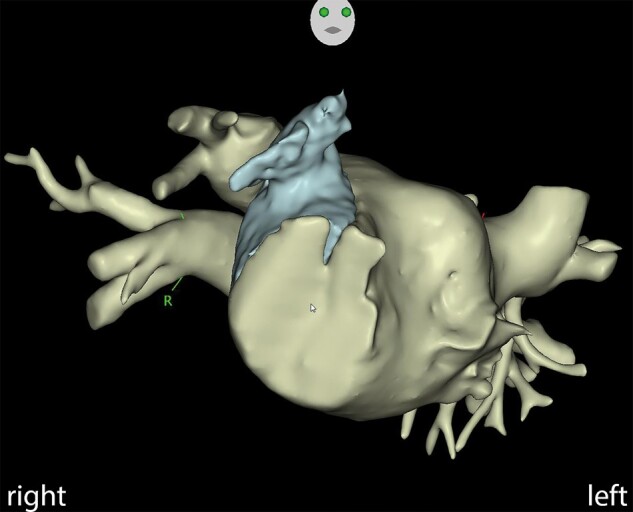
3D reconstruction of cardiac CT, showing the systemic atrium and atrial appendage (RAO view).
